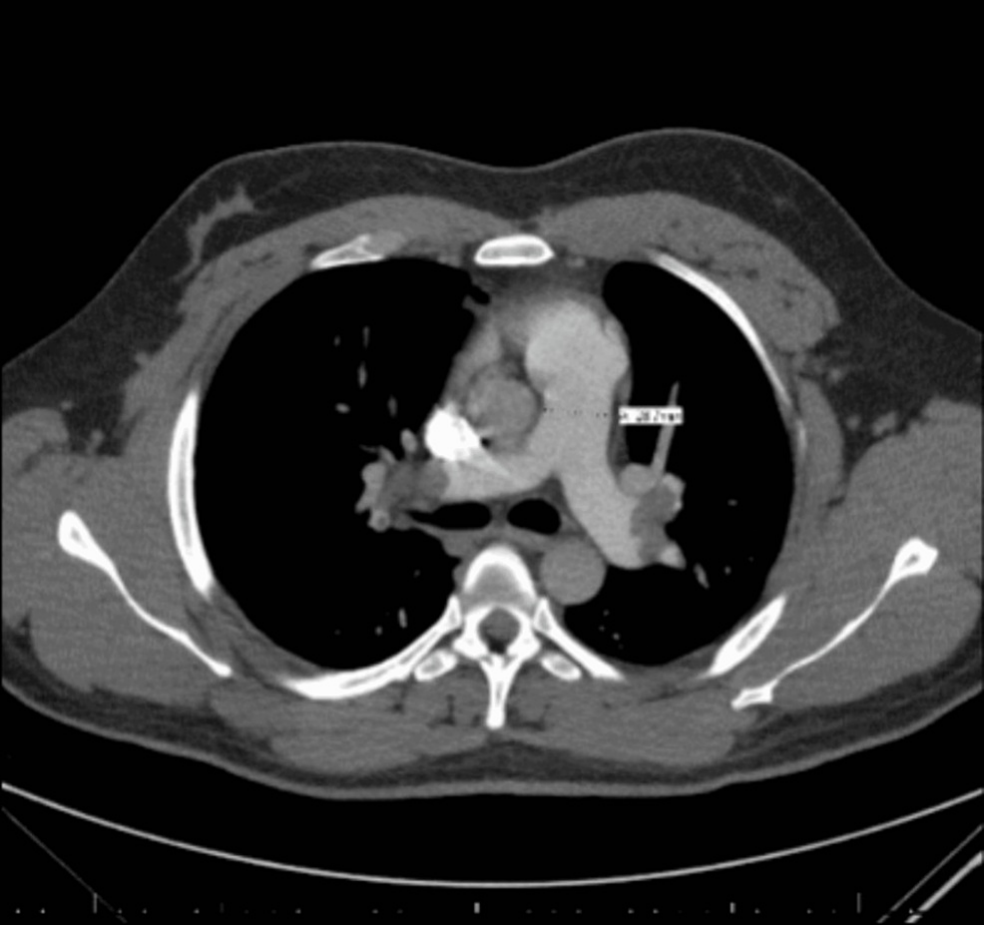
Chest CTA Showing Multiple Pulmonary Emboli CTA: CT angiography
